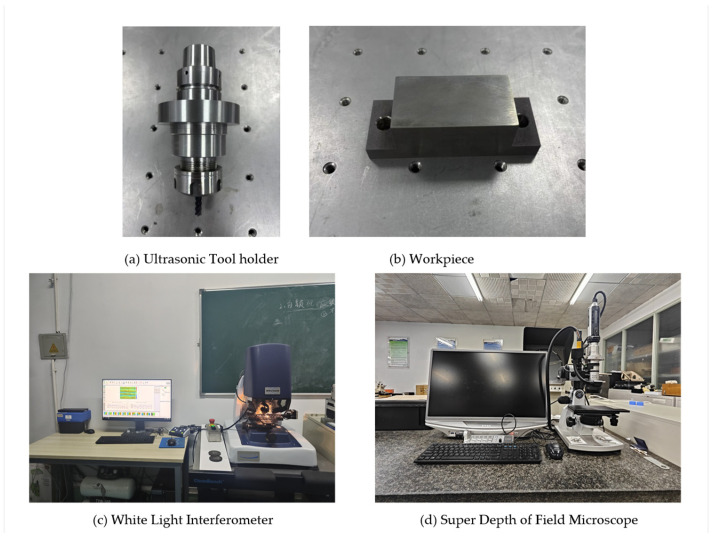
Ultrasonic tool holder and workpiece. Measurement equipment used in the experiment.
